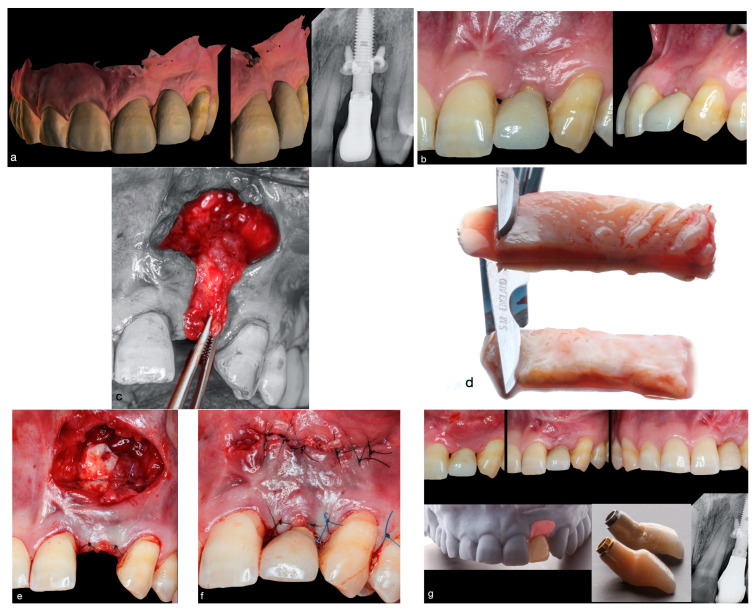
(a) Initial clinical situation. Periapical radiograph showing an excessively apical implant position. (b) Condition after modifying the cervical–buccal contour of the implant-supported crown. As well as the deficient MT, the partial loss of both papillae can be observed. (c) Dissection of the connective tissue pedicle graft. (d) Graft before de-epithelialization and graft after de-epithelialization with a 15C blade. (e) A CTG harvested from the palate was sutured over the connective tissue pedicle graft. (f) Flap closure. Suspension stitches were used in the marginal zone and simple stitches were placed without much tension to approximate the edges of the horizontal apical incision. (g) Healing 2 weeks (when stitches were removed), 1 month, and 4 months postsurgery (note the scar on the apical alveolar mucosa resulting from the surgical access incision). A cement- and screw-retained zirconia restoration with full reduction and ceramic stratification.
